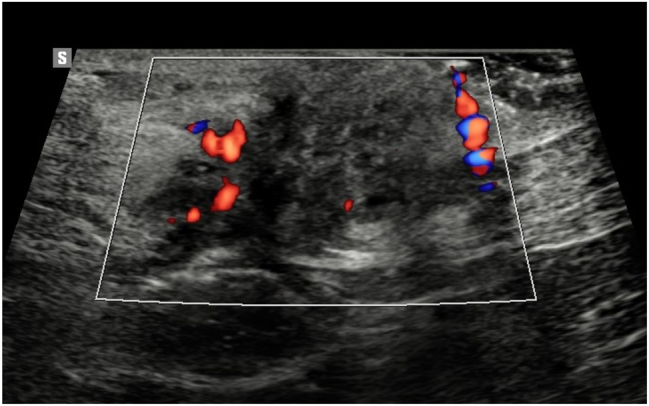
Ultrasound image of the umbilical mass demonstrating a solid, heterogeneous lesion with peripheral vascularization on Doppler, consistent with characteristics of abdominal wall endometriosis.
